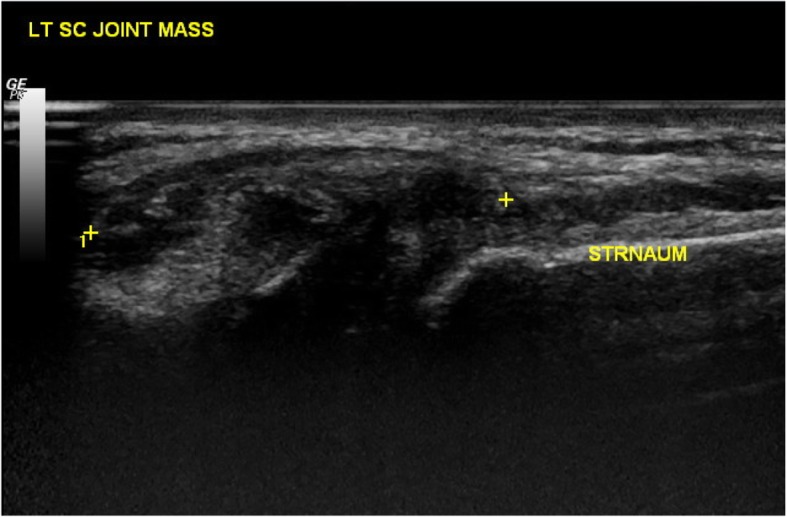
Ultrasonography of the left sternoclavicular joint shows a heterogeneous hypoechoic mass in a 14-year-10-month-old male patient with juvenile idiopathic arthritis
